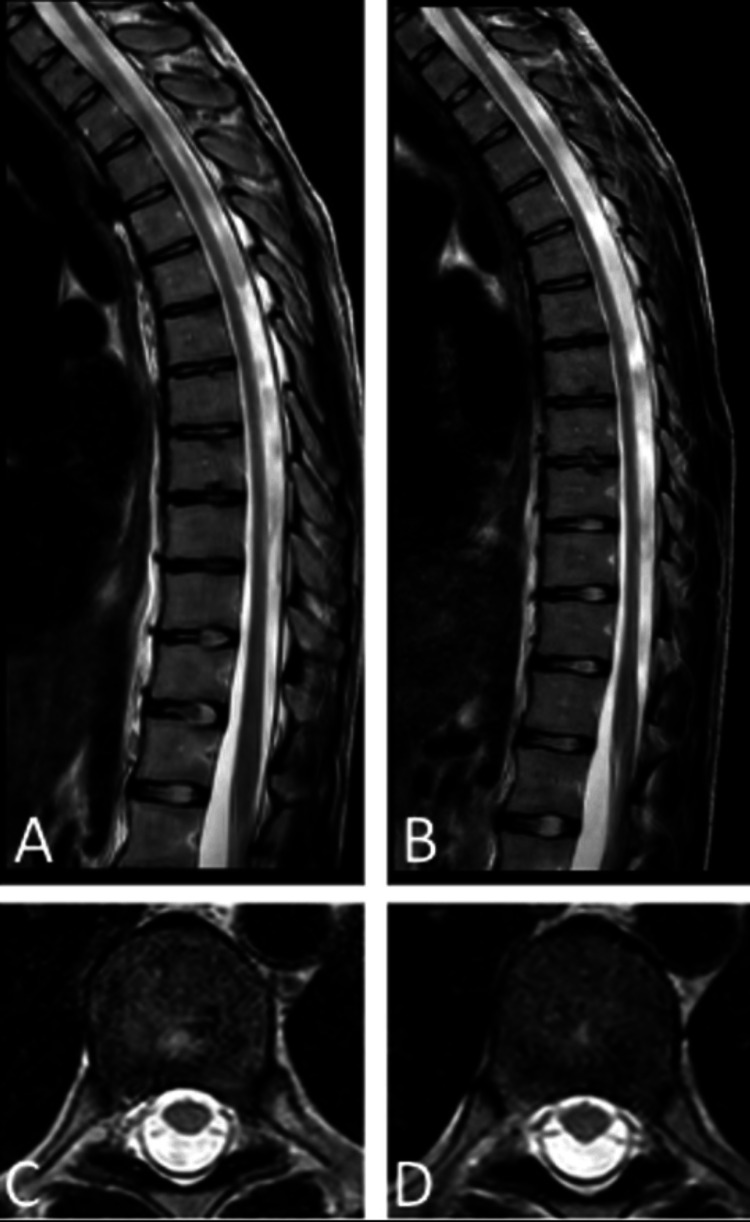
Thoracic spinal cord MRI of the twin patients. Thoracic spine MRI, sagittal T2 (A) and (B) consistent with atrophy. Axial sequences (C) and (D) at the level of the T9 vertebral body. Patient A (A) and (C) and patient B (B) and (D).
